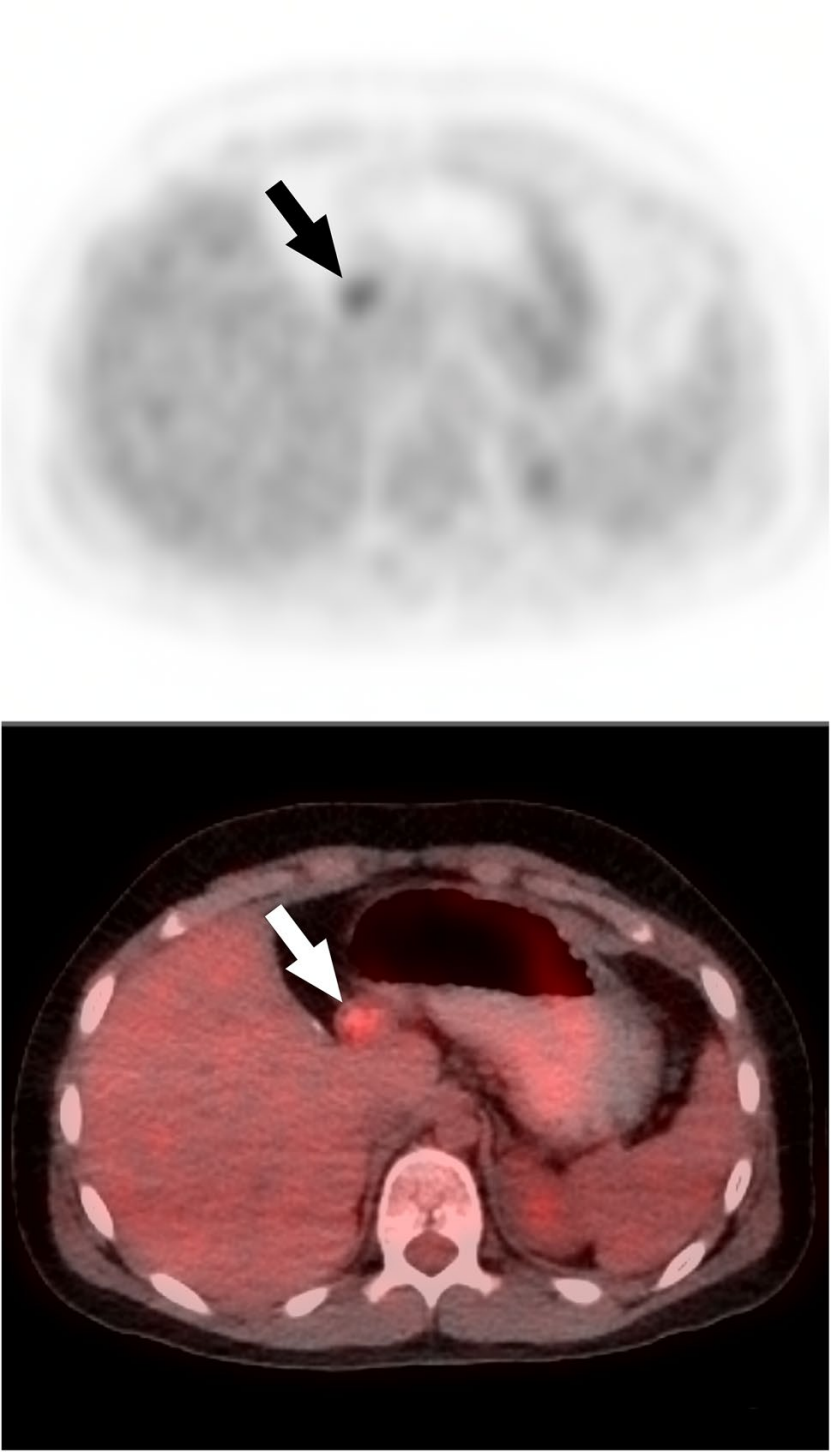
Axial PET and fused PET/CT images from ^18^F-FDG PET/CT in an 11-year-old boy with hepatoblastoma. The primary interpretation reported an “area of FDG activity along the pylorus… metastatic implants not excluded…” and recommended further diagnostic MRI or CT. The secondary interpretation reported “no findings of recurrent or metastatic disease” and “upper abdominal activity described on the outside report reflects activity associated with the pylorus and is not concerning for disease.” *Arrows* indicate the finding
